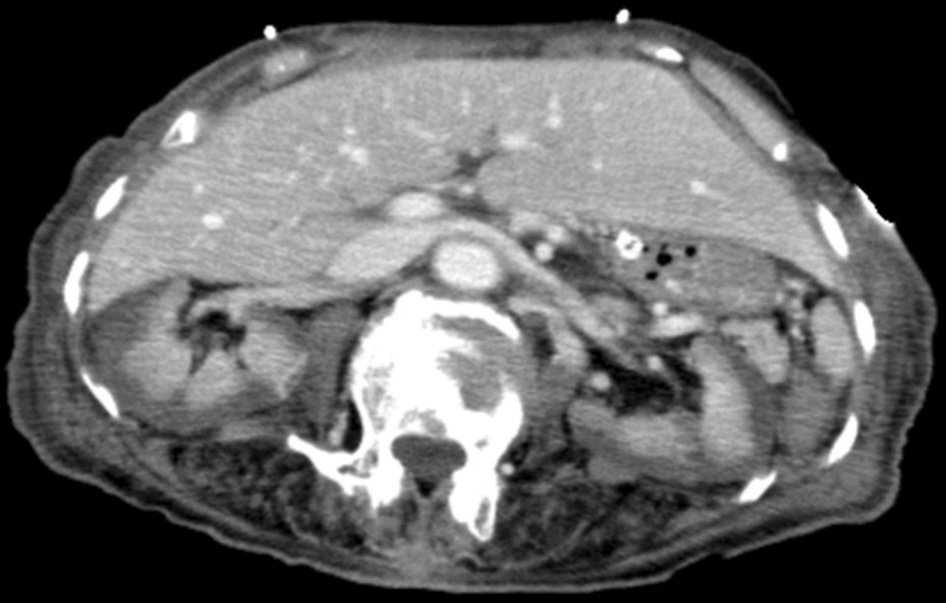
Contrast-enhanced computed tomography in the axial view demonstrating a non-enhancing renal cortex against a background of intact medullary perfusion
